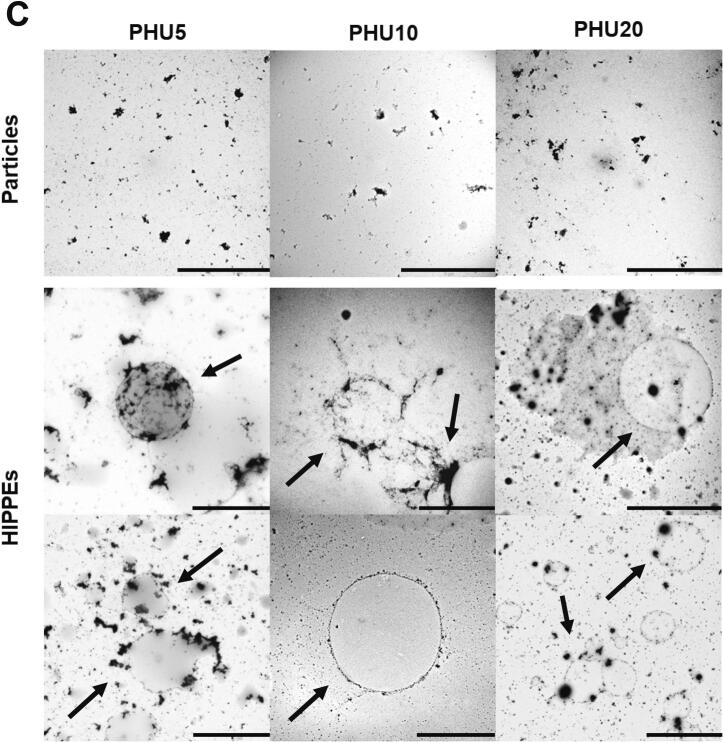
TEM images of legume protein particles and HIPPEs. HIPPEs stabilized by untreated protein isolates (FCON and PCON) (A), HU-treated FBPI particles and the corresponding HIPPEs (B), and HU-treated PPI particles and the corresponding HIPPEs (C). HIPPE, high internal phase Pickering emulsion; FBPIs (F), faba bean protein isolates; PPIs (P), pea protein isolates; CON, untreated 4 % protein dispersions; HU10, 4 % protein dispersions sequentially heated and ultrasonicated for 10 min; Scale bar = 10 μm.
